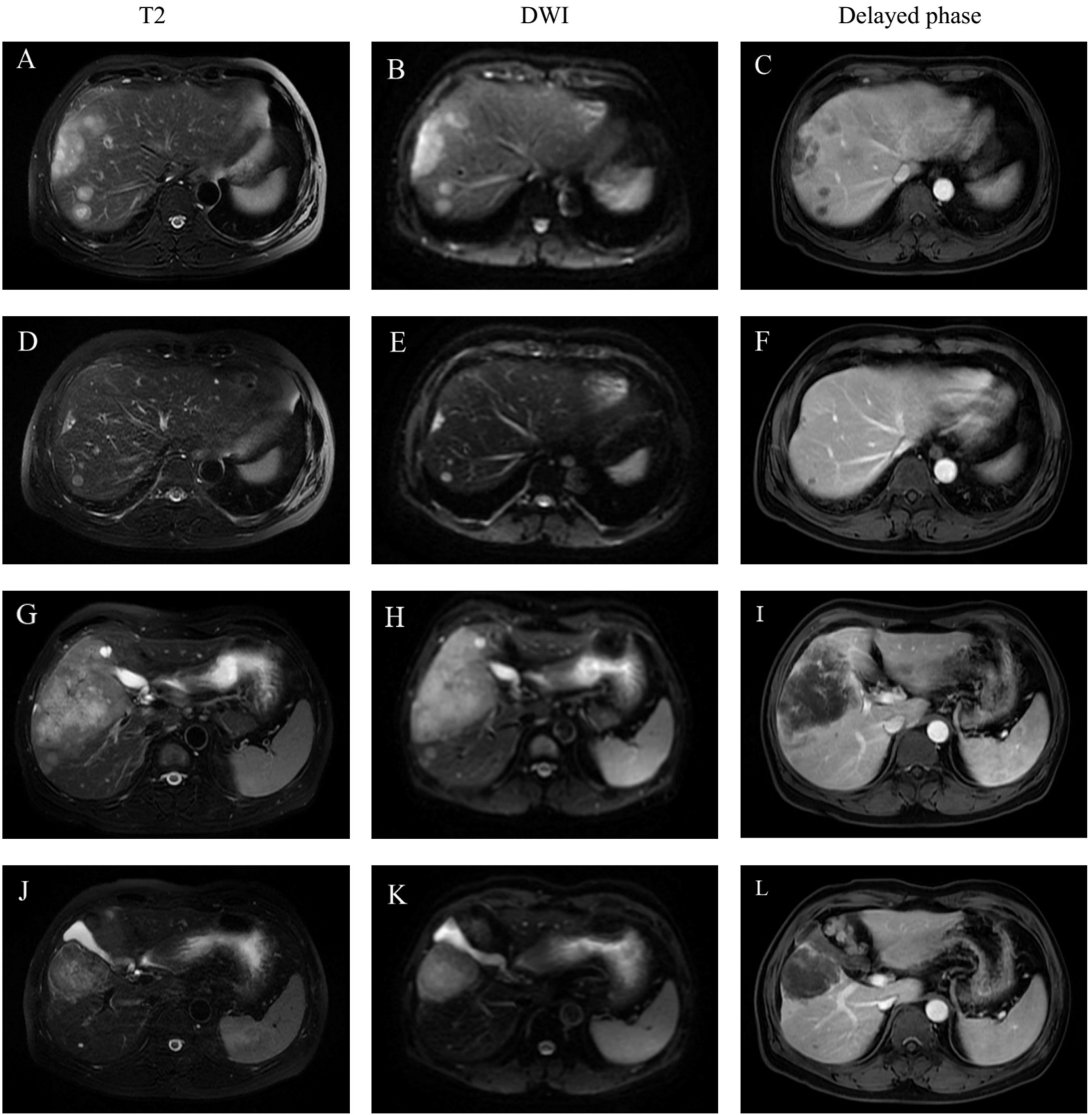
Representative MR imaging revealed the changes of multiple lesions before and after combination treatment. (A, D, G, J): An axial T2-weighted MR image. (B, E, H, K): An axial diffusion weighted image (DWI). (C, F, I, L): An axial delayed phase images. (A, B, C: A dynamic contrast-enhanced MRI showed that multiple liver tumor located in the segment 7 and 8 before treatment. (D, E, F: MRI results after six cycles of treatment revealed that the tumor located in the segment 7 and 8 has shrunk in diameter and the number of tumors decreased. (G, H, I): MRI showed the largest tumor (measuring 11.5 cm in diameter) located in the right anterior lobe and segment 6 before treatment. (J, K, L): The diameter of the largest liver lesion located in the right anterior lobe and segment 6 significantly decreased from 11.5cm to 7.7cm after six cycles of treatment. There was no markedly enhancement within the tumor during the delay phase.
